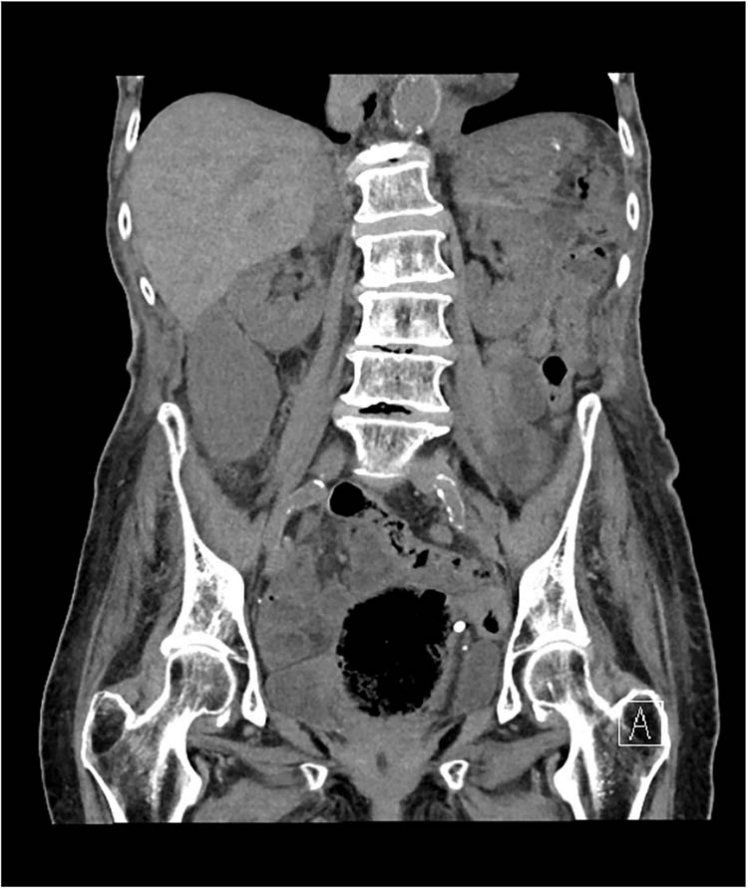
Axial slice of non-contrast CT abdomen and pelvis, demonstrating a distended gallbladder located outside the typical anatomical location, inferior the liver edge, causing medial displacement of the right kidney.
